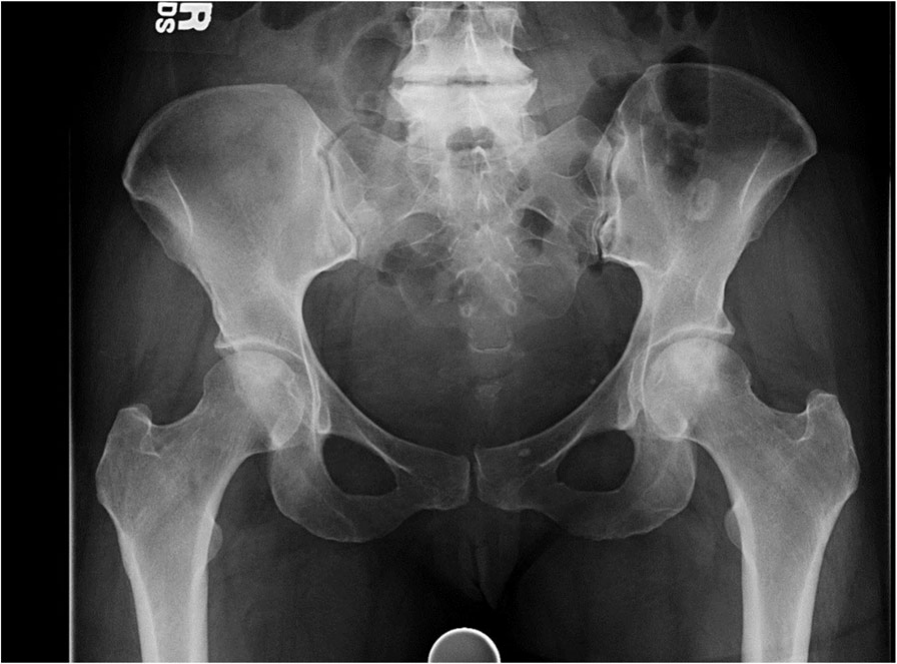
Plain AP pelvis X-ray at 5 years follow-up of a patient with a Stage IIa lesion of the left hip that shows preserved joint space without lesion progression
